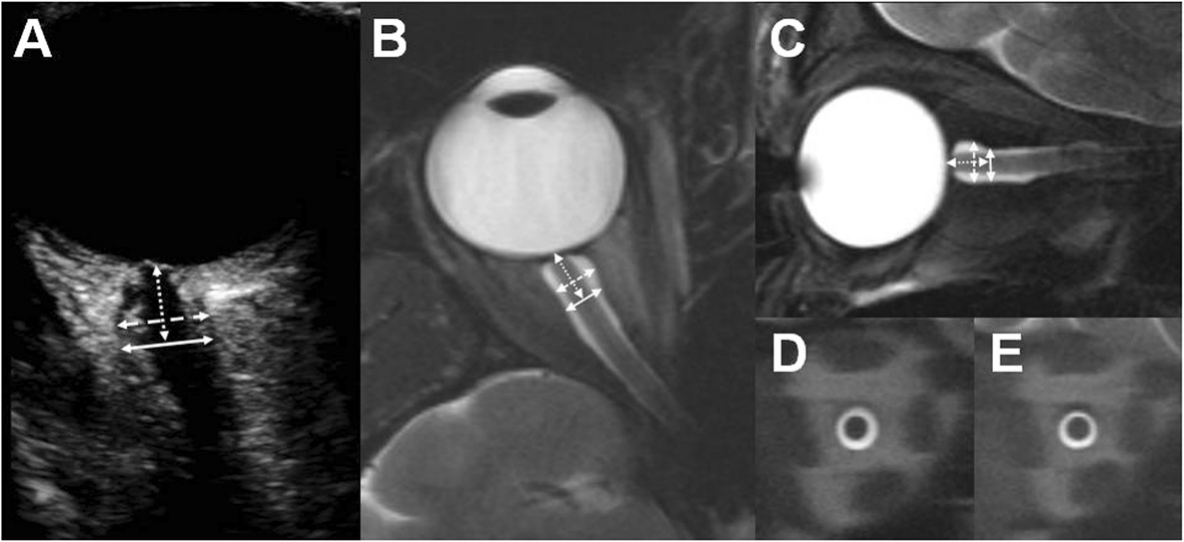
Transbulbar sonography and MRI of the optic nerve sheath. (A) The eye bulb and the optic nerve are displayed by B-mode sonography. The optic nerve sheath diameter was measured 3 and 5 mm behind the papilla (dotted arrow) in an axial plane showing the optic nerve in its longitudinal course. Transverse (B) and sagital (C) T2-weighted TSE sequences were created to schedule coronal and orthogonal HASTE sequences of the optic nerve sheath 3 mm (D) and 5 mm (E) behind the papilla.
